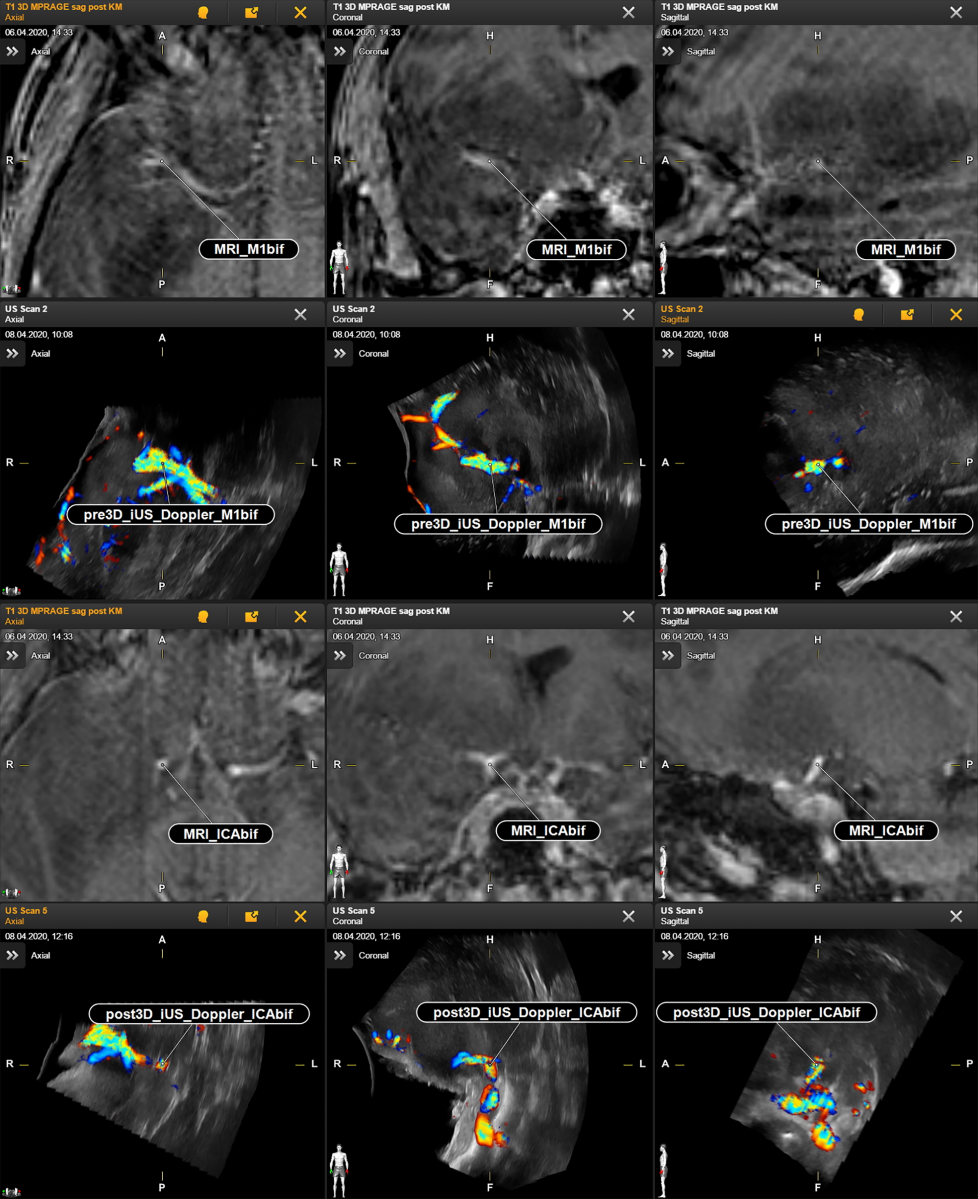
Case no. 2. Identification of vascular anatomic landmarks in MRI and navigated reformatted 3D iUS Doppler. First and second row: preoperative MRI and corresponding pre-resectional 3D iUS Doppler in axial, coronal, and sagittal plane. Third and fourth row: preoperative MRI and corresponding post-resectional 3D iUS Doppler in axial, coronal, and sagittal plane. CAbif, internal carotid artery bifurcation; M1bif, M1bifurcation; pre3D iUS Doppler, pre-resectional 3D iUS Doppler; post3D iUS Doppler, post-resectional 3D iUS Doppler.
